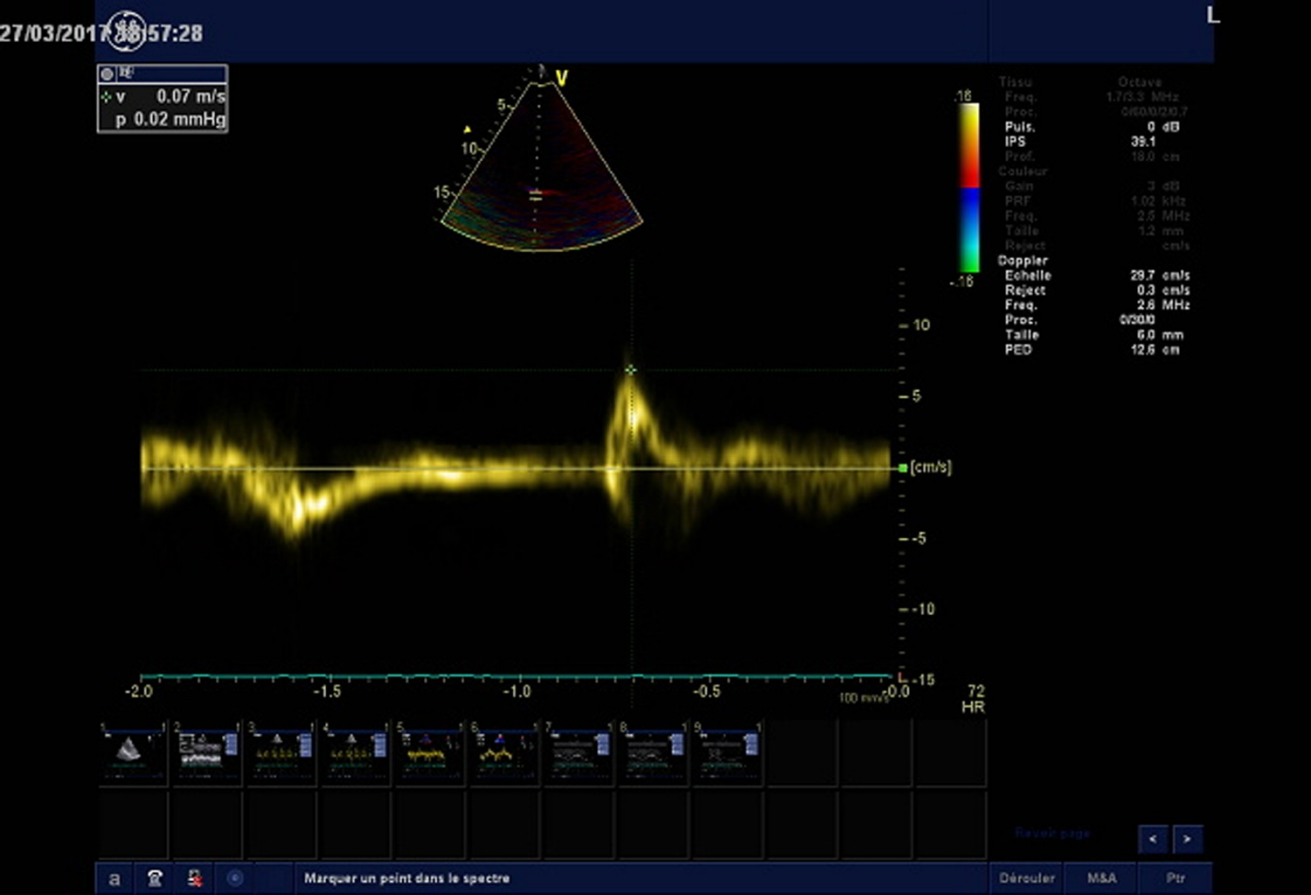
Pathological right hemidiaphragm peak tissue Doppler imaging velocity during a sniff maneuver in a DMD patient. Note the reduced peak sniff diaphragm TDI velocity (arrow).
